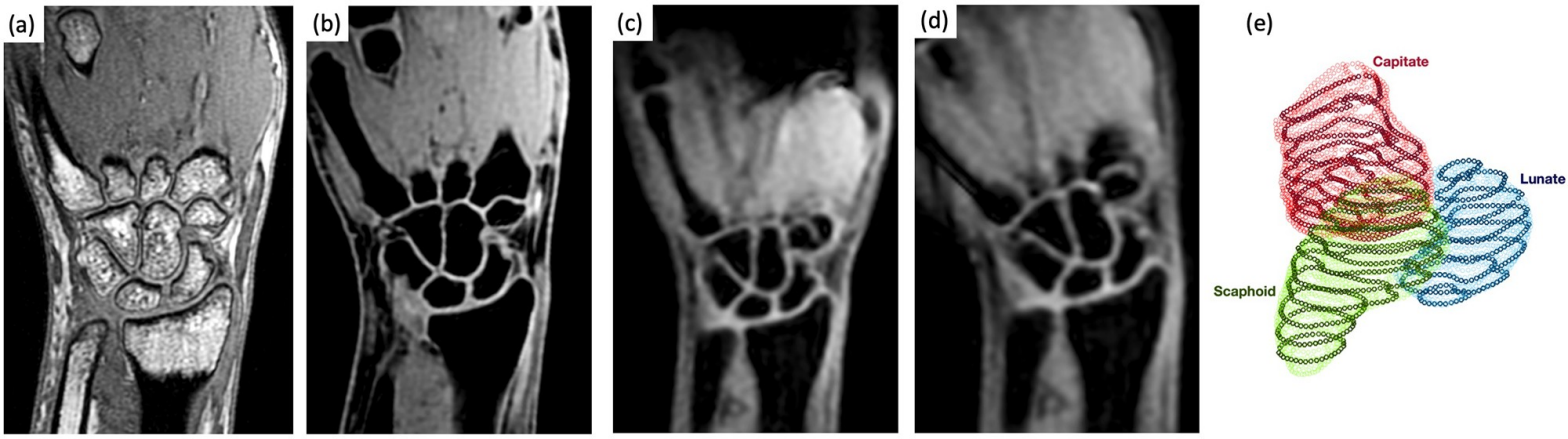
Sample static and dynamic MR images and registered point clouds. Orthogonal imaging planes through the carpal bones of (a) 3D SPGR and (b) LAVA Flex acquisitions. LAVA Flex water-decomposed images were used manually segment the bones of interest. (c,d) Sample slices of 3D dynamic LAVA Flex images utilized to track the static segmentation. (e) Resulting point cloud of boundary points derived from the high-resolution segmentation. The thick points show the boundaries of the moving slices which are registered to the static point clouds.
